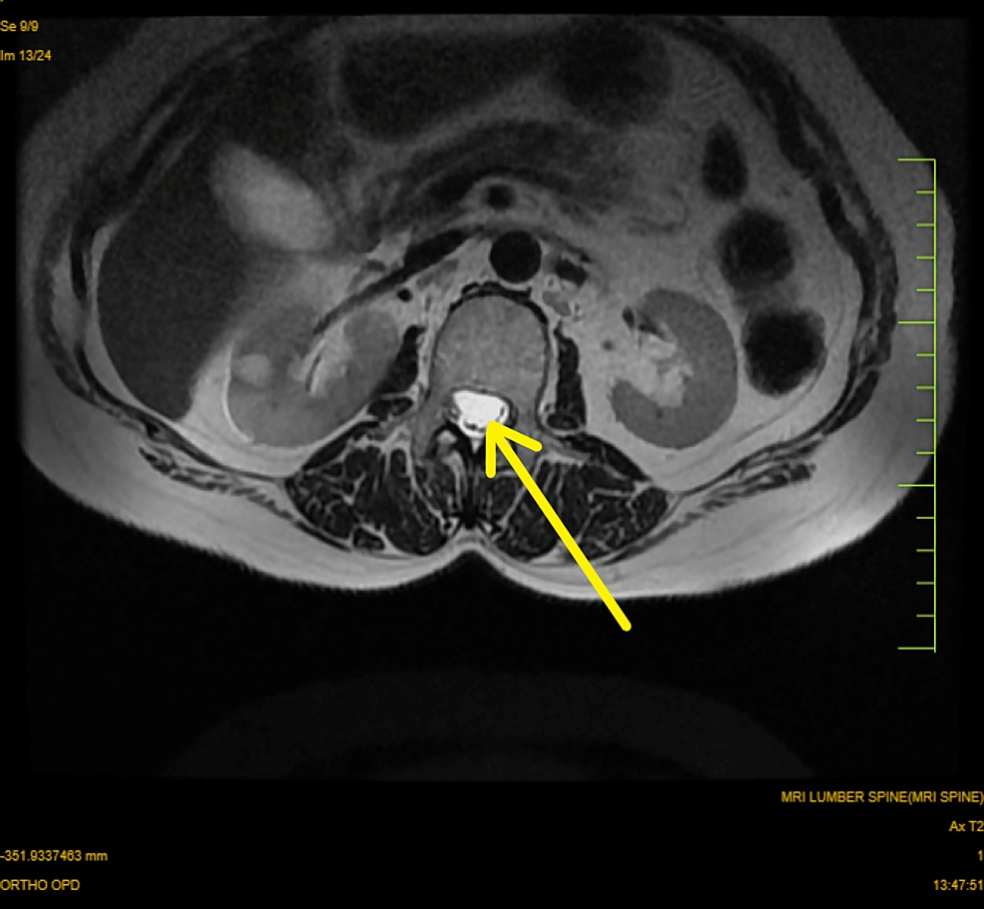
MRI (transverse section) The yellow arrow is showing the tumor
